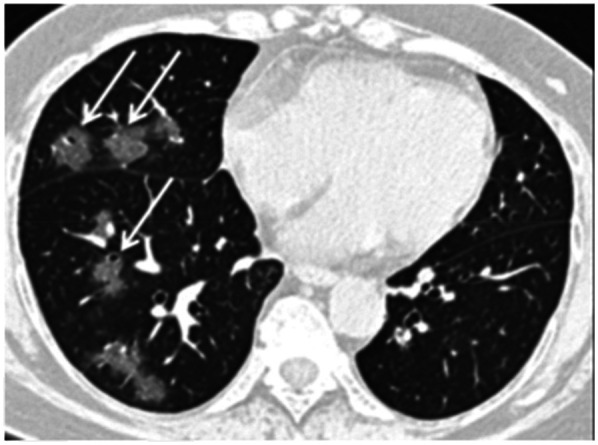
Unenhanced axial CT image from 56‐year‐old woman shows ground‐glass opacities with rounded morphology (arrows) in right middle and lower lobes. Left lung was normal. (Reprinted with permission of (32).)
